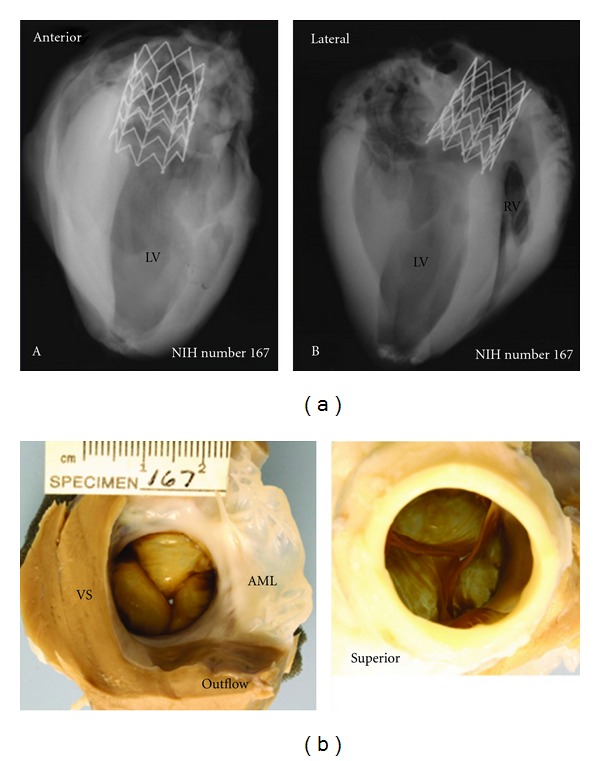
Radiographs (a) and necropsy results (b) of the hearts with the self-expanding prosthesis 6-months postimplantation. Both anterior and lateral views of the heart show an intact self-expanding stent frame without any fractures. The bottom row shows inferior and superior views of the self-expanding prosthetic aortic prosthesis; note that the entire stent frame crowns are covered as well as the annulus of the prosthetic valve is covered by opaque white tissue without pannus formation extending into the valve bases. The anterior mitral leaflet is unremarkable. The superior view of the aortic prosthetic valve shows good coaptation of the free edges of the valve leaflets. VS-ventricular septum, AML-anterior mitral leaflet.
